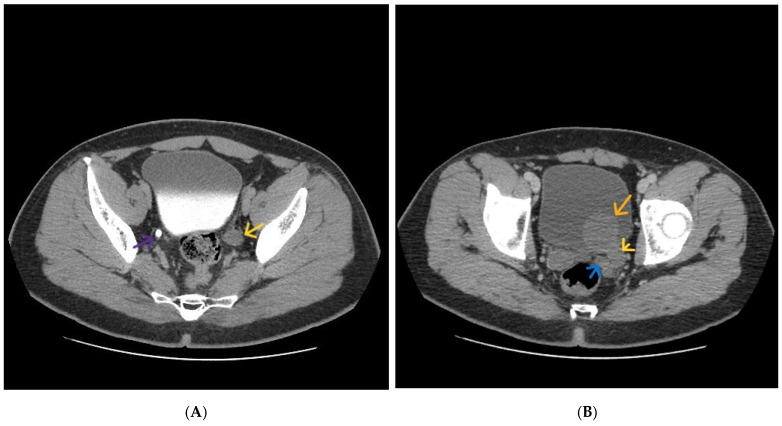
(A) axial CT image, delayed phase, showing dilated left ureter (yellow arrow). Normal right ureter (purple arrow). (B) axial CT image. Dilated ejaculatory duct (blue arrow). Left ureterocele (orange arrow). Dilated left ureter (yellow arrow).
